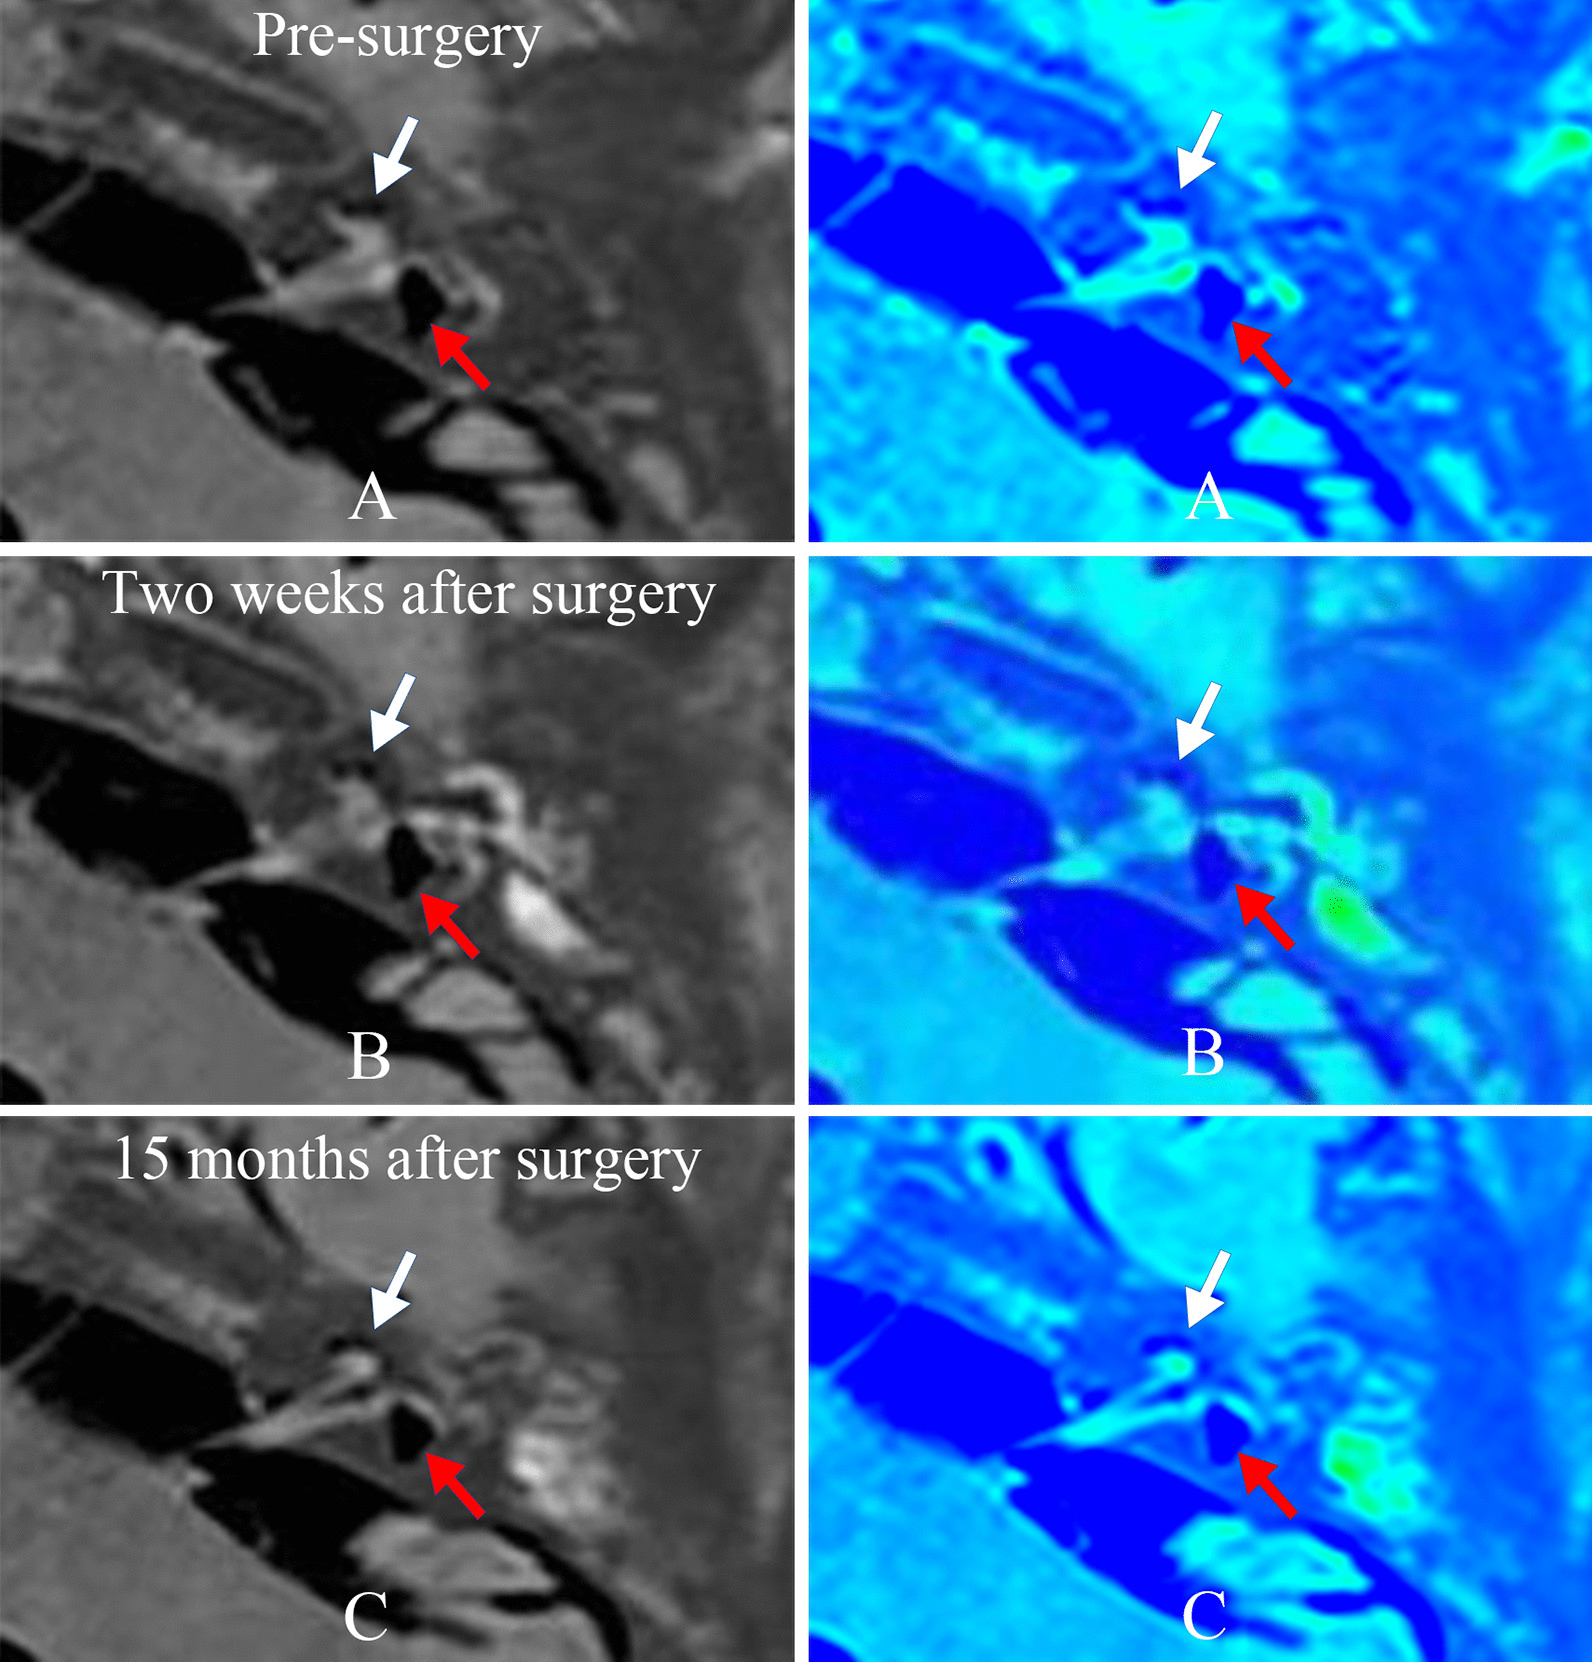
A Gd-MRI (left column) and the corresponding MRI colored images (right column) showed a significant cochlear (white arrow) and vestibular hydrops (red arrow) in the left ear of patient no. 23 (Table 1) before surgery. Postoperative images showed no changes of cochlear EH (white arrow) and vestibular EH (red arrow) both 2 weeks (B) and 15 months (C) following ESD surgery comparing with pre-surgery recording. *Gd-MRI* gadopentetate dimeglumine magnetic resonance imaging, *EH* endolymphatic hydrops, *ESD* endolymphatic sac decompression, *MRI* magnetic resonance imaging
